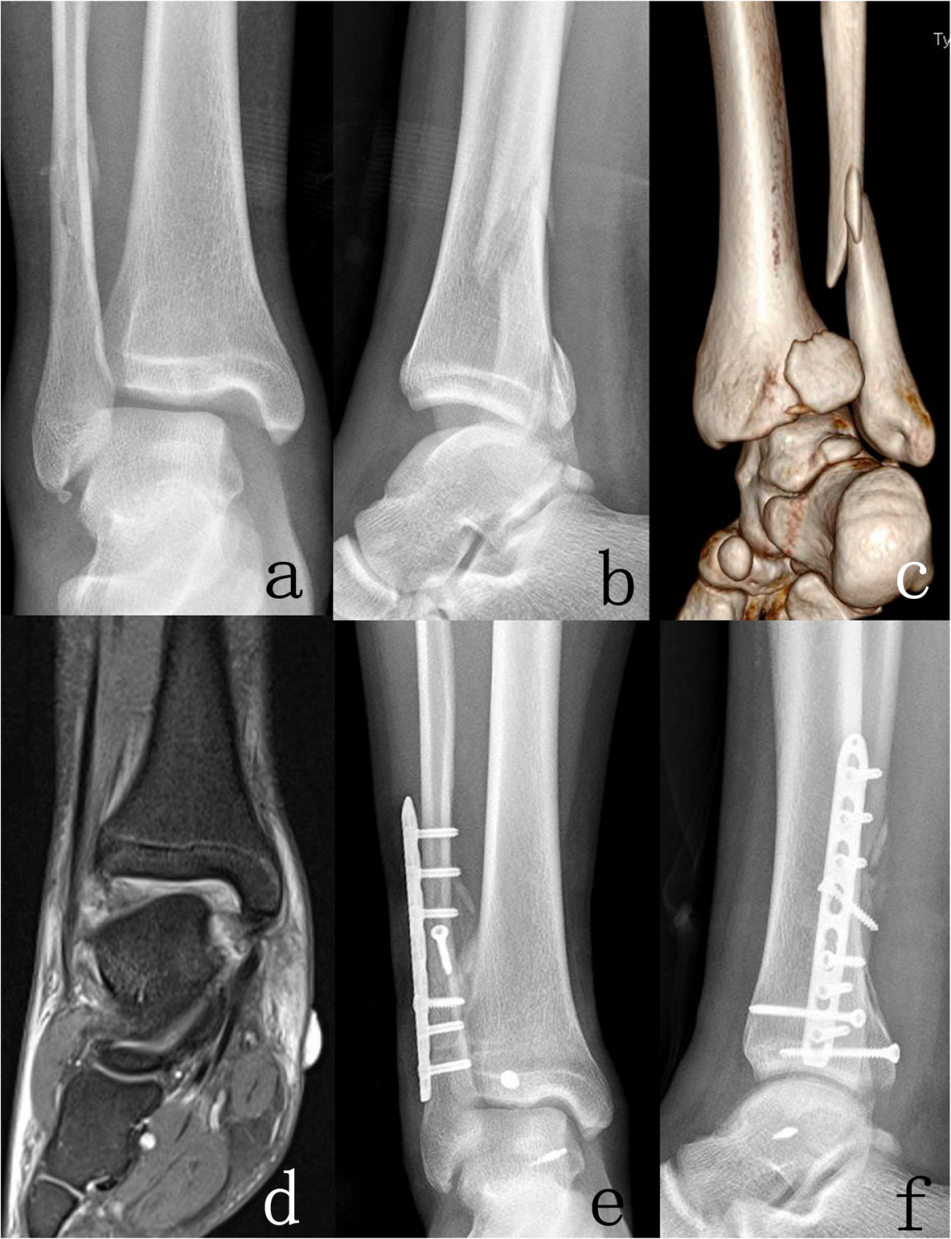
Imaging studies of a 17 years patient with SER IV ankle fractures (AO/OTA classification 44-B3.1) underwent ORIF with DLR. a-c Preoperative X-rays and three-dimensional CT. d Preoperative MRI showing deltoid ligament injury. e, f Postoperative anteroposterior and lateral X-rays
